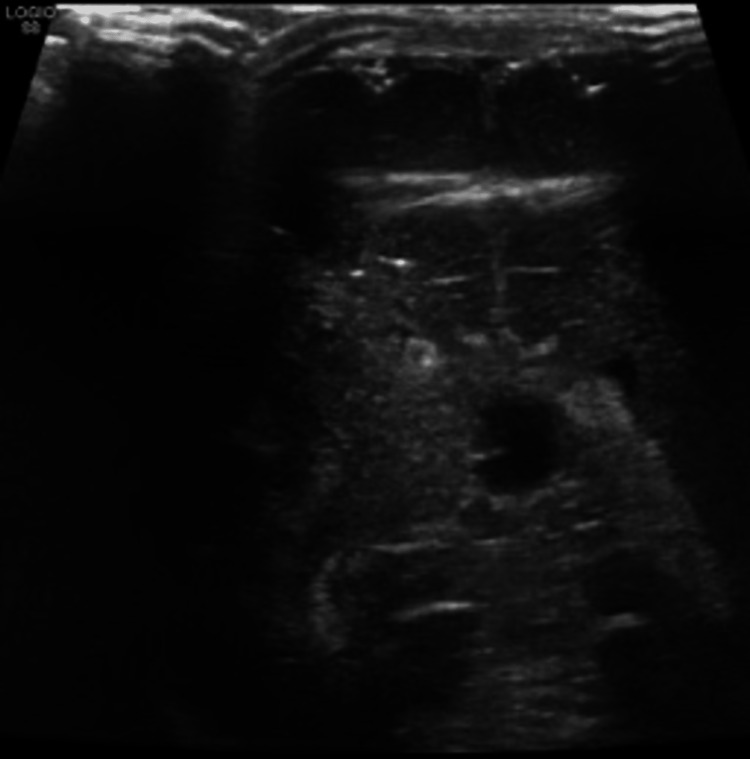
Transcranial ultrasound (transverse view) showing diffuse hypoechoic appearance of the brain parenchyma with indistinct gray-white matter interface and symmetrical ventricular system attenuation. Scattered innumerable hypoechogenic foci. No signs of intracranial hemorrhage.
